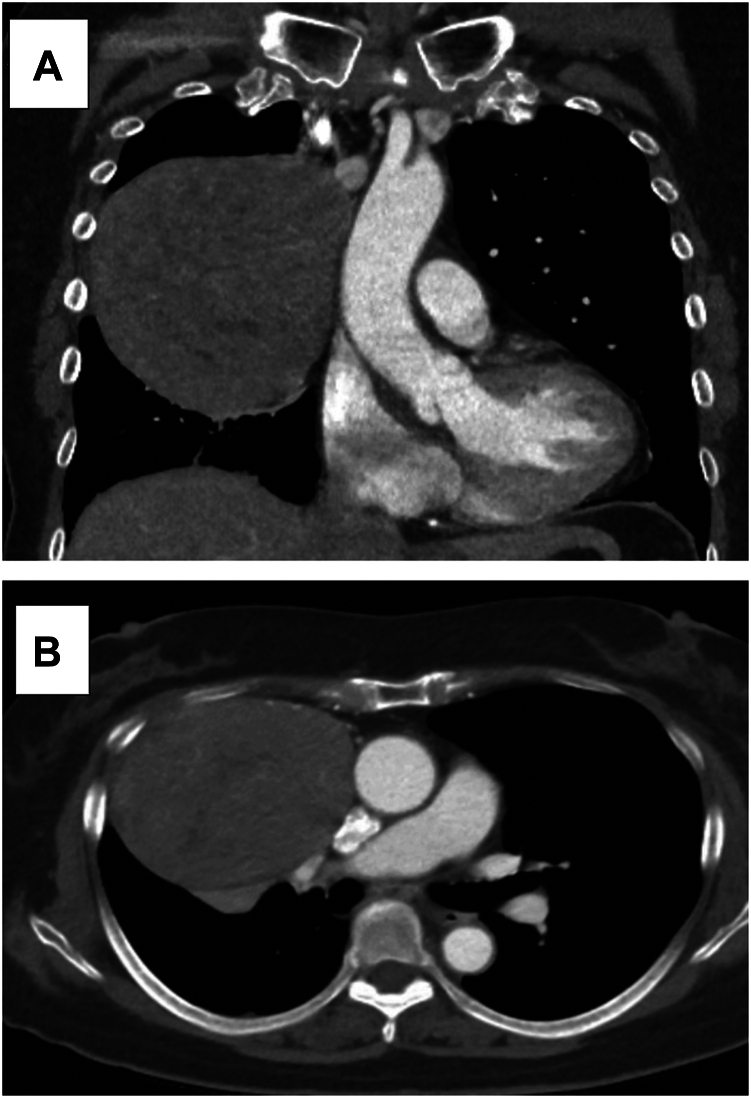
Preoperative computed tomography imaging of the thymoma. (A) Coronal view. (B) Axial view.
